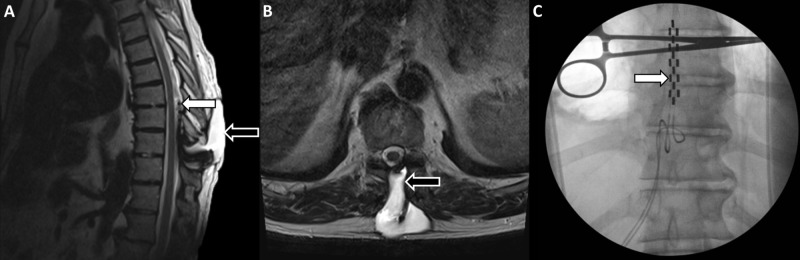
Imaging studies A: preoperative T2 sagittal MRI shows a pseudomeningocele (black arrow) and a spinal cord stimulator paddle (white arrow); B: preoperative T2 axial MRI redemonstrates the pseudomeningocele (black arrow); C: intraoperative fluoroscopic imaging shows the spinal cord stimulator paddle (white arrow) MRI: magnetic resonance imaging
